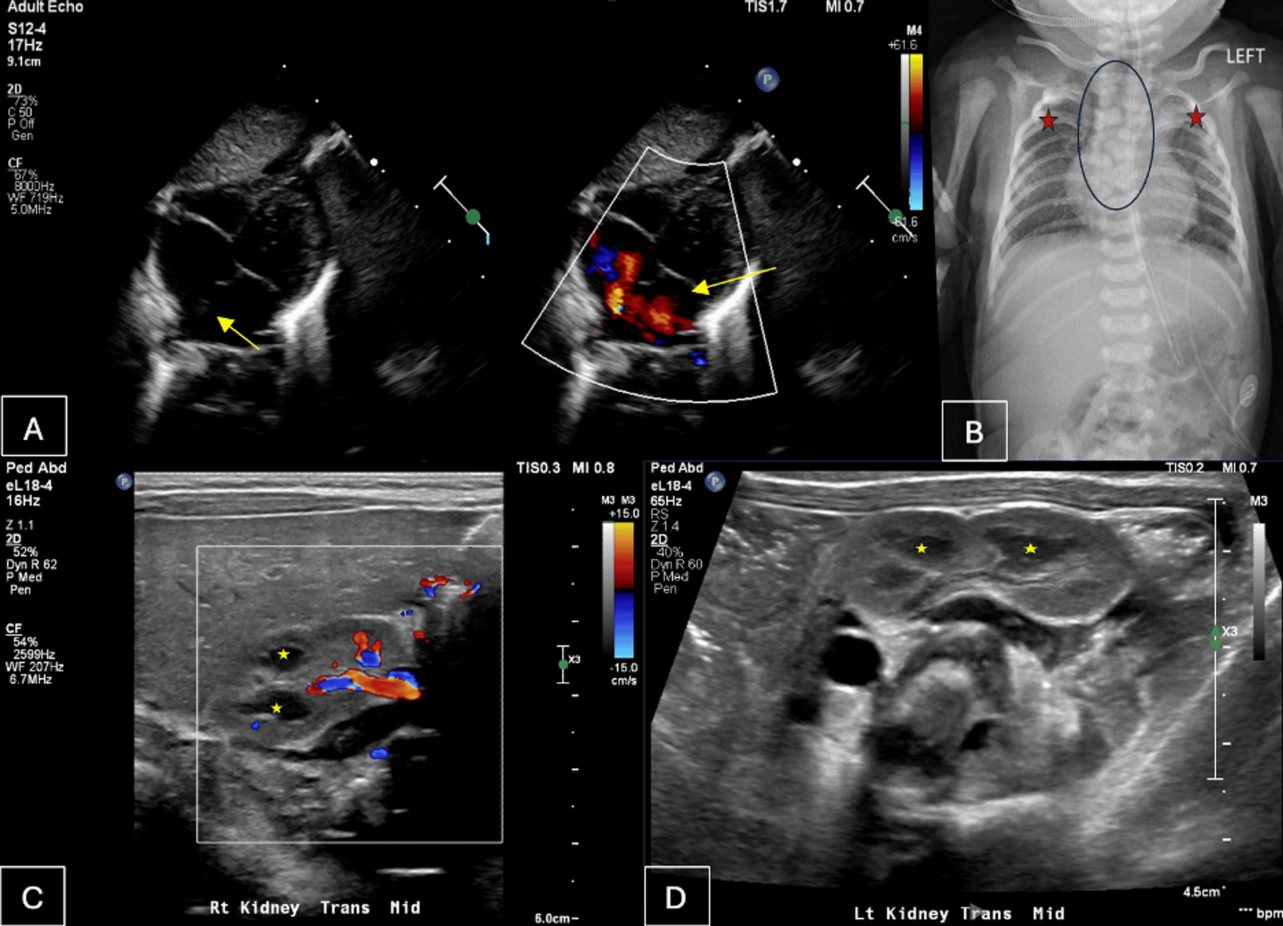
VACTERL imaging findings (A) Two-dimensional (2D) echocardiography of the patient showing a small to moderate-sized secundum type atrial septal defect (yellow arrow) with left to right shunt noted by the red-colored flow across the atrial septal defect (ASD). (B) Frontal view chest X-ray of the patient showing multiple thoracic vertebral anomalies (black circle) in addition to distortion of ribs bilaterally (red asterisks). Pediatric abdominal ultrasound of the patient with a transverse view of the (C) right kidney and (D) left kidney showing bilateral renal calyceal dilation (yellow asterisks) without significant dilation of the renal pelvis, most consistent with congenital megacalyces. Hilar vessels are indicated by the color Doppler (C). ASD, atrial septal defect; VACTERL: vertebral defects, anal atresia, cardiac defects, tracheoesophageal fistula or atresia, renal anomalies, and limb abnormalities
